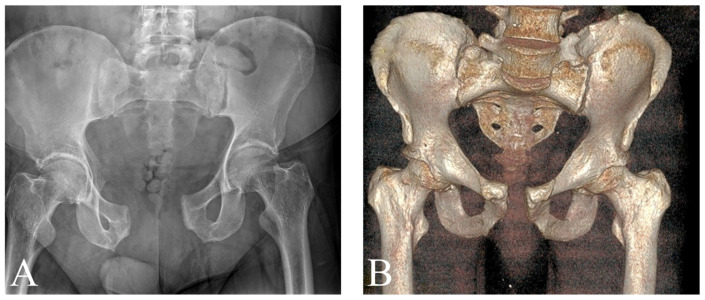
AP pelvic X-ray of Patient 3 upon arrival (A) and CT scan after placement of the binder (B). The X-ray shows AO/OTA 61B3.3 injury; the disrupted symphysis measures 60 mm. After application of the binder, the width of the symphysis reduces to 16 mm, and both sides of the SI joint seem anatomically aligned.
